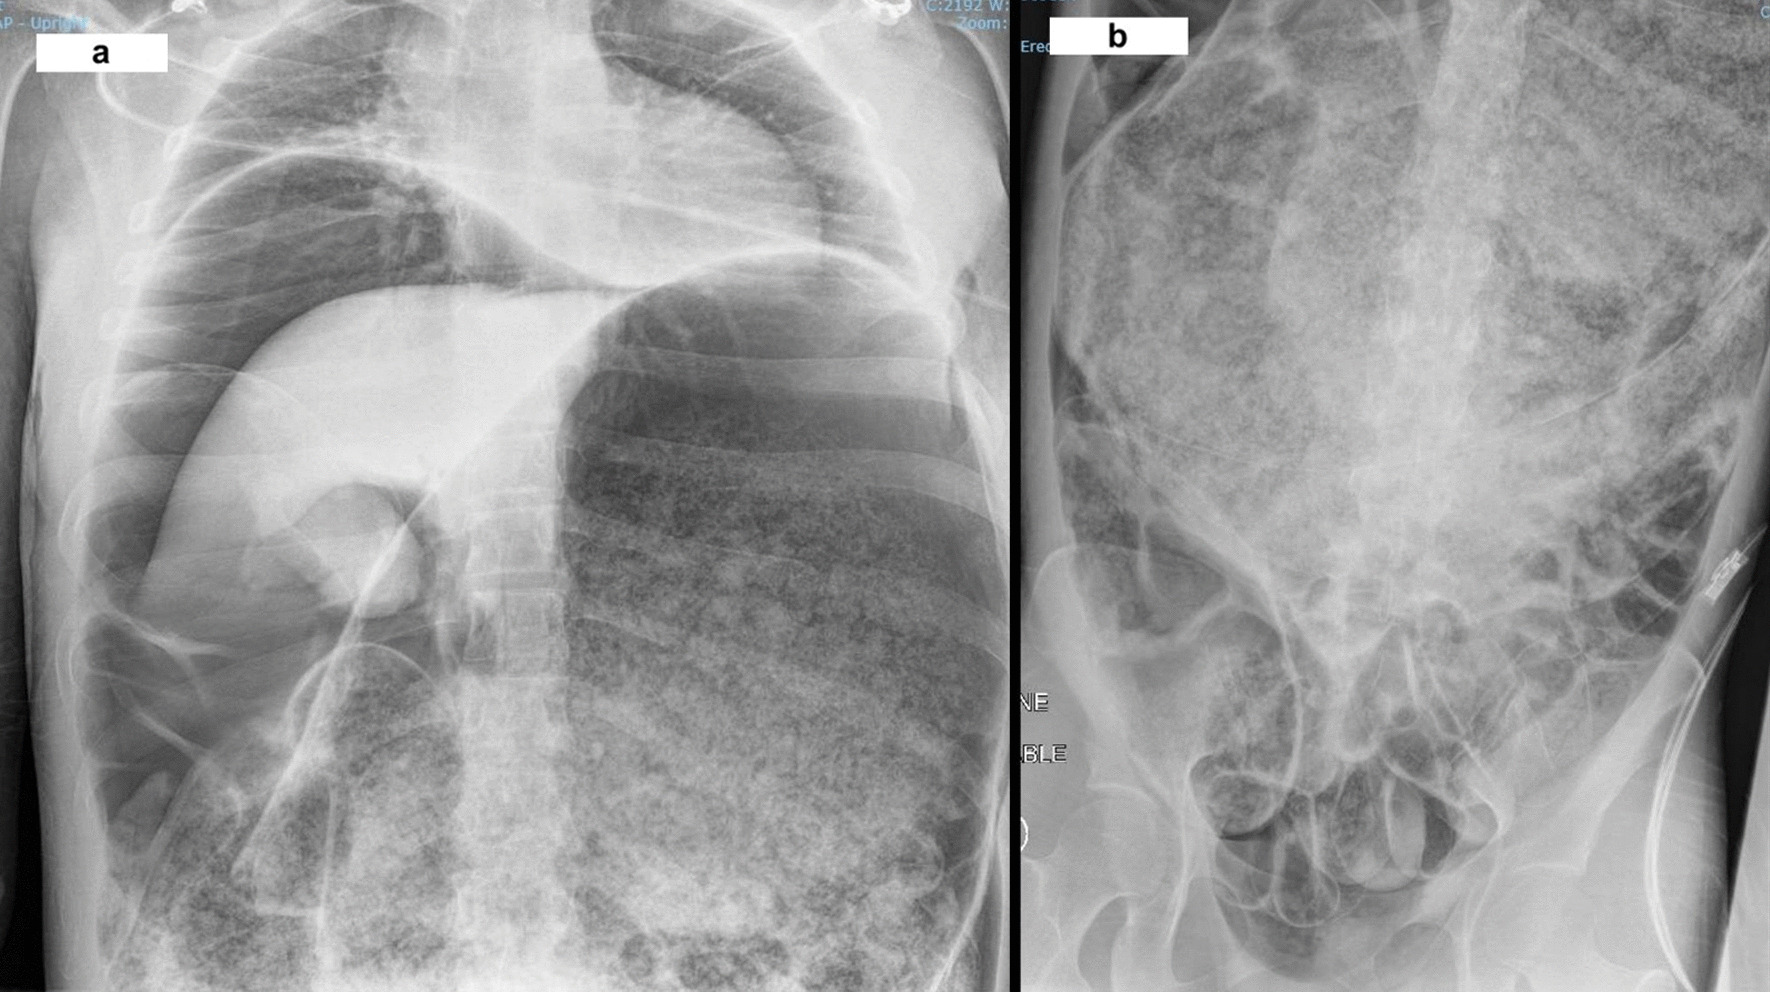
a Portable X-ray of chest showing pneumoperitoneum and markedly dilated stomach. b Portable X-ray of abdomen showing grossly dilated stomach to pelvis
